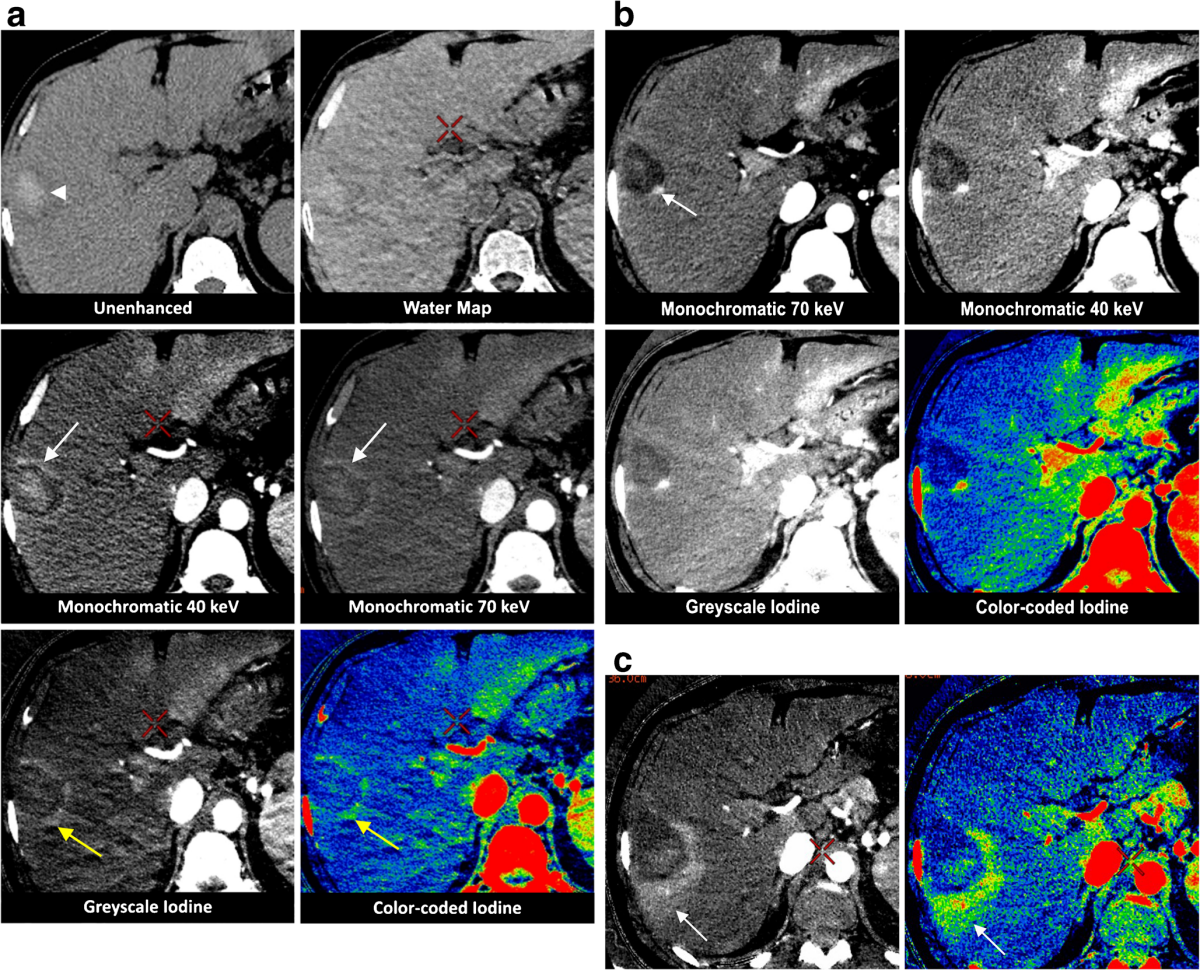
Hepatocellular carcinoma. A 43-year-old male with cirrhosis undergoes gadolinium enhanced MRI, revealing a hepatocellular carcinoma in segment VII, typical for a hepatocellular carcinoma, which is confirmed through biopsy. a Twenty-four hours after RF ablation, the patient undergoes a standard unenhanced CT scan and a contrast-enhanced DECT. The unenhanced CT shows a central area of high attenuation (*arrowhead*) within the ablation zone, which has a lower visibility on the water map reconstruction. The central hyperdensity is well noticeable on the synthesised monochromatic 40-keV but less so on the 70-keV images, and it is hardly visible on the iodine images. On the 40- and 70-keV synthesised monochromatic images, the ablation zone can be depicted as a lesion with a hypervascular peripheral rim (*white arrows*). On the greyscale- and colour-coded iodine images, we can better appreciate the focal hypervascular thickening at the posterior border (*yellow arrows*) than with the synthesised monochromatic images. b Due to the suspicious nature of the focal thickening seen in a, a shorter follow-up period is adhered to and a DECT is performed 5 weeks post-RF ablation. A focal hypervascular nodule is well depicted on the 70-keV (*white arrow*) images, although more clearly observable on the 40-keV ones; however again it is more evident on the greyscale- and colour-coded iodine images. Biopsy of this region confirms residual HCC. c Twenty-four hours after the second ablation, the greyscale- and colour-coded iodine images show significant iodine rim uptake around the ablation zone in the arterial phase (*arrows*). Therefore, a focal hypervascular remnant cannot be appreciated. This rim disappears on the venous phase (not shown). MRI after 10 weeks reveals no signs of reactivation
